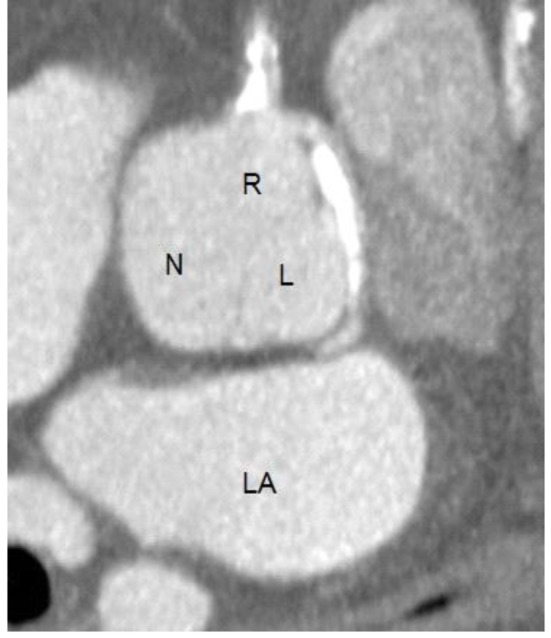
Coronary CT angiogram showing the right (R), left (L) and non-coronary (N) cusps. The right coronary cusp bares the ostia of the right coronary artery as well as the left coronary artery. The left atrium (LA) is also visualized.
